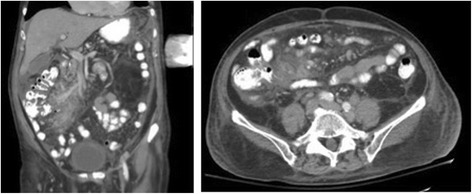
Computed tomography of the abdomen and pelvis. Coronal and axial computed tomography images with contrast of the patient’s abdomen showing extensive retroperitoneal, pelvic, and mesenteric lymphadenopathy in addition to extensive peritoneal and omental thickening with ascites
